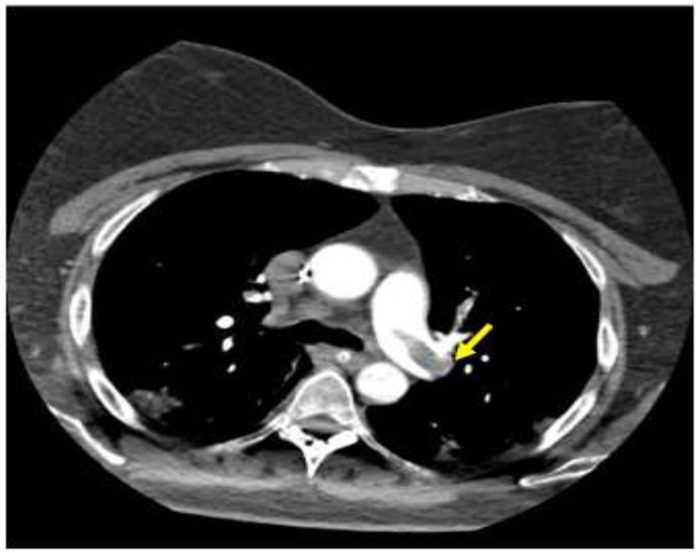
Computed tomography scan imaging of acute respiratory distress syndrome (ARDS) associated with COVID-19 and pulmonary emboli: pulmonary embolus across the bifurcation of the pulmonary trunk is noted, as indicated by the arrow.
